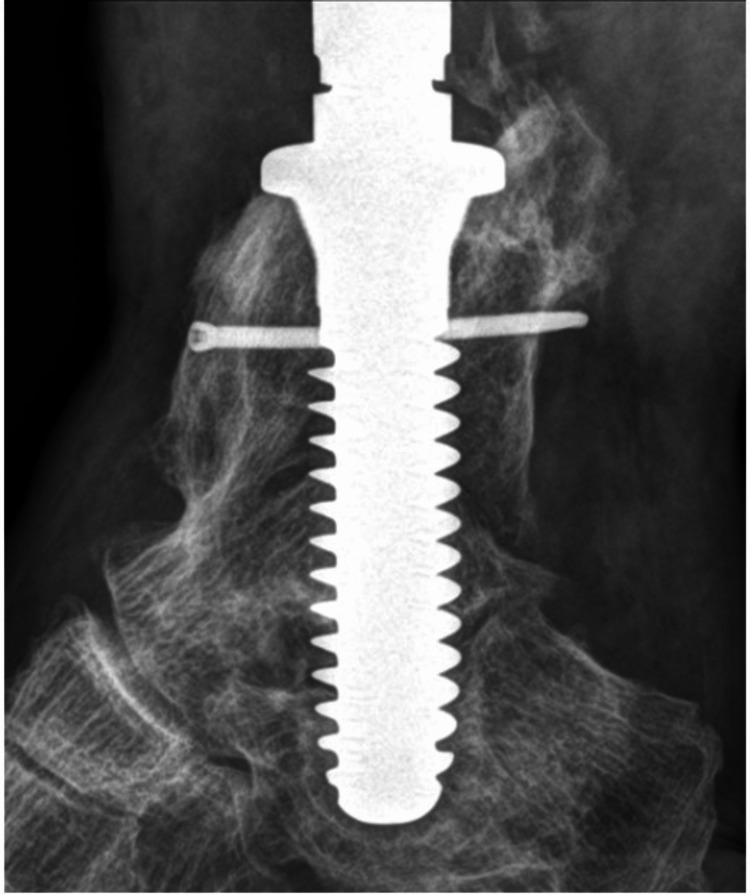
Radiograph demonstrating the stability of the stem at 2.5 years after implantation.
